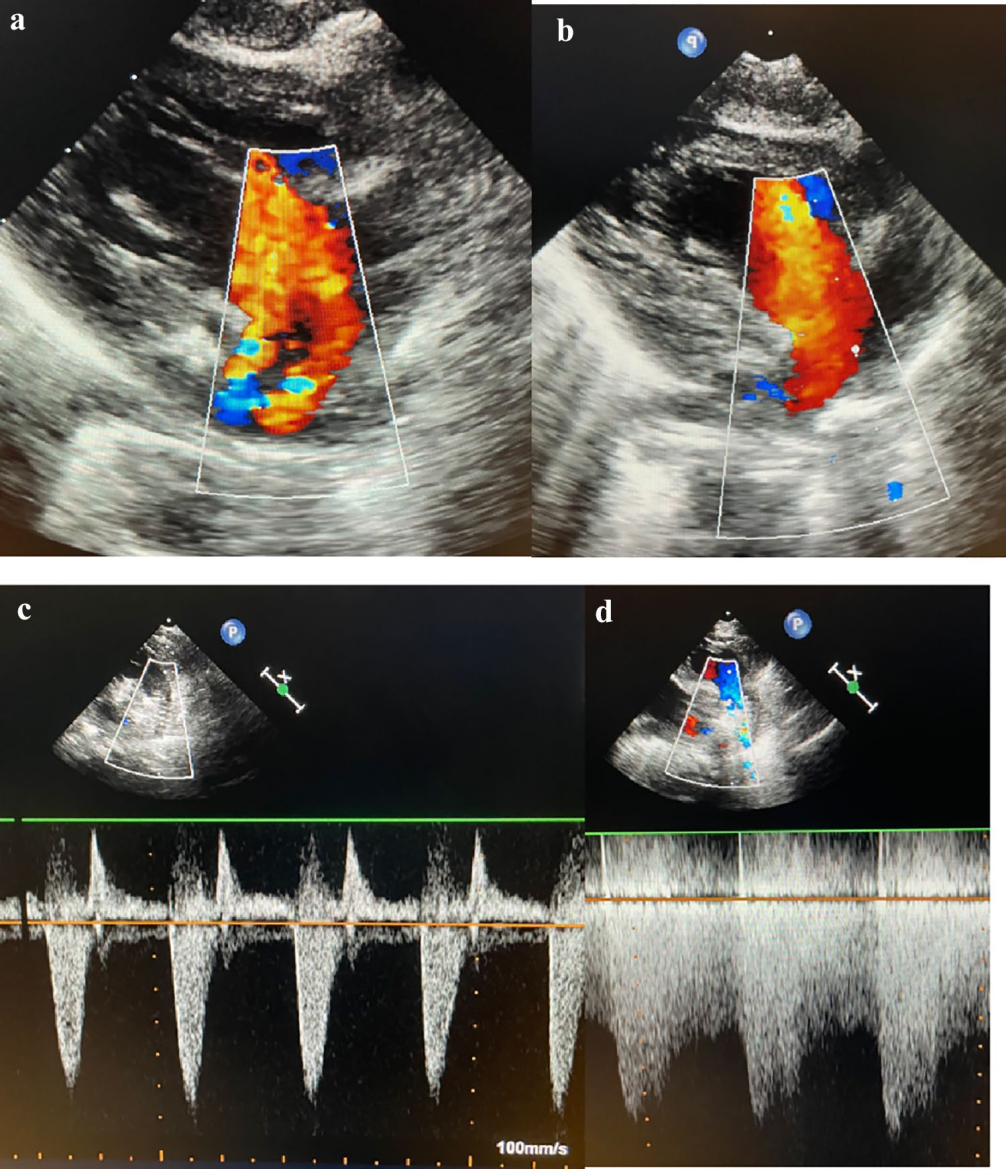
Percutaneous S1P: echocardiographic documentation. a The interatrial communication of a multiperforated atrial septum is shown with two red-colored left-to-right jets. The diminished left ventricle with a hypoplastic mitral valve is adumbrated. b An unobstructed atrial communication after Rashkind procedure is shown with laminar red-colored flow from left atrium through ruptured atrial septum through the tricuspid valve. c CW-Doppler is shown with adequate systolic right-to-left through the stented arterial duct with an acceptable diastolic left-to-right shunt component due to PFRs in the pulmonary artery branches. d Shows a systolic-diastolic CW-Doppler pattern corresponding to highly efficient endoluminal banding of the LPA by a PFR
